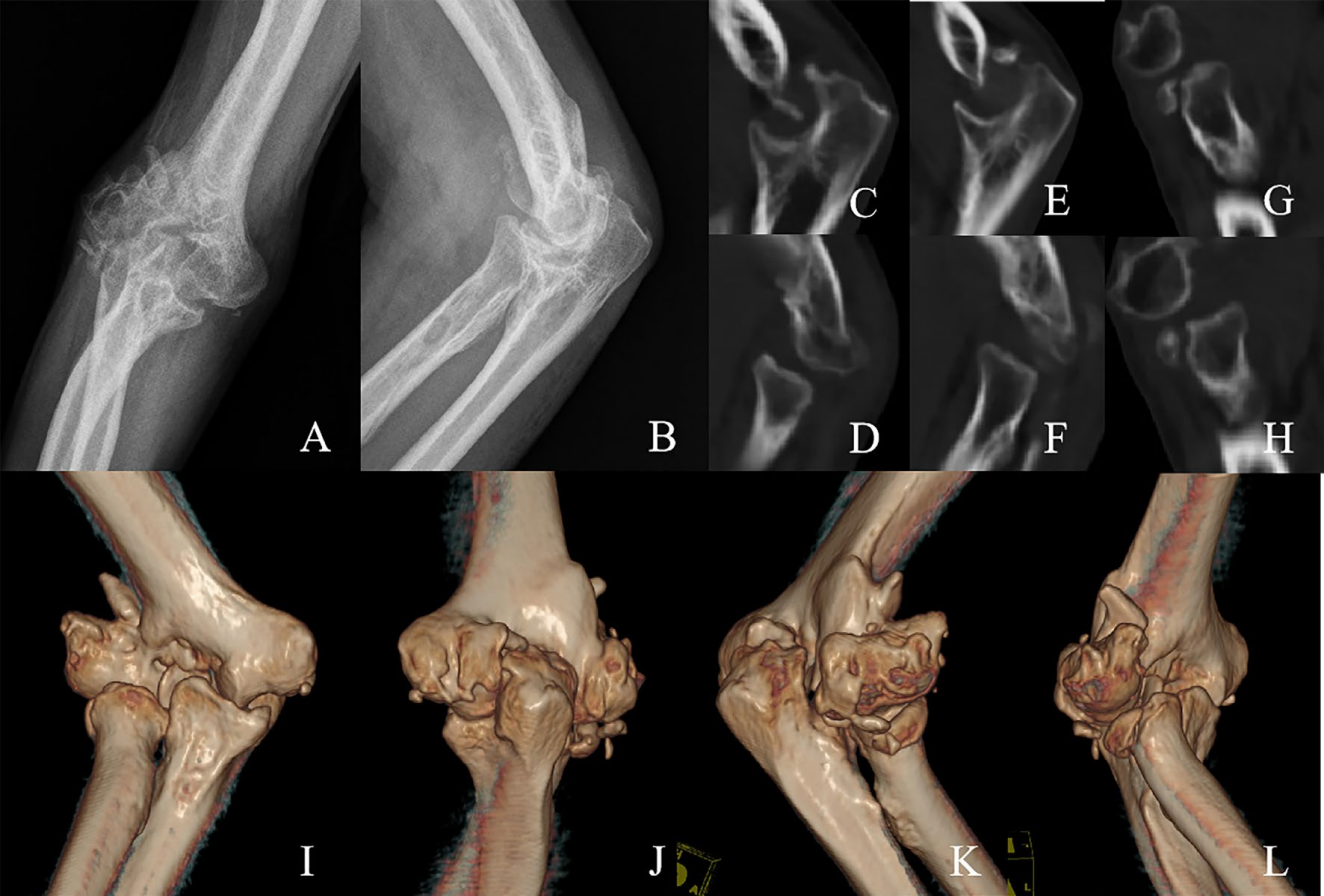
(A and B) Anteroposterior and lateral view of the injured elbow joint demonstrated bony deformity of the distal humerus. (C–F) The articular surface at the proximal ulna and radius presented with mild joint degeneration. (G and H) Nonunion of the radial head fracture with minimal displacement and articular step‐off. (I–L) 3D‐CT scan revealed grievous deformed distal humerus and heterotopic ossification.
